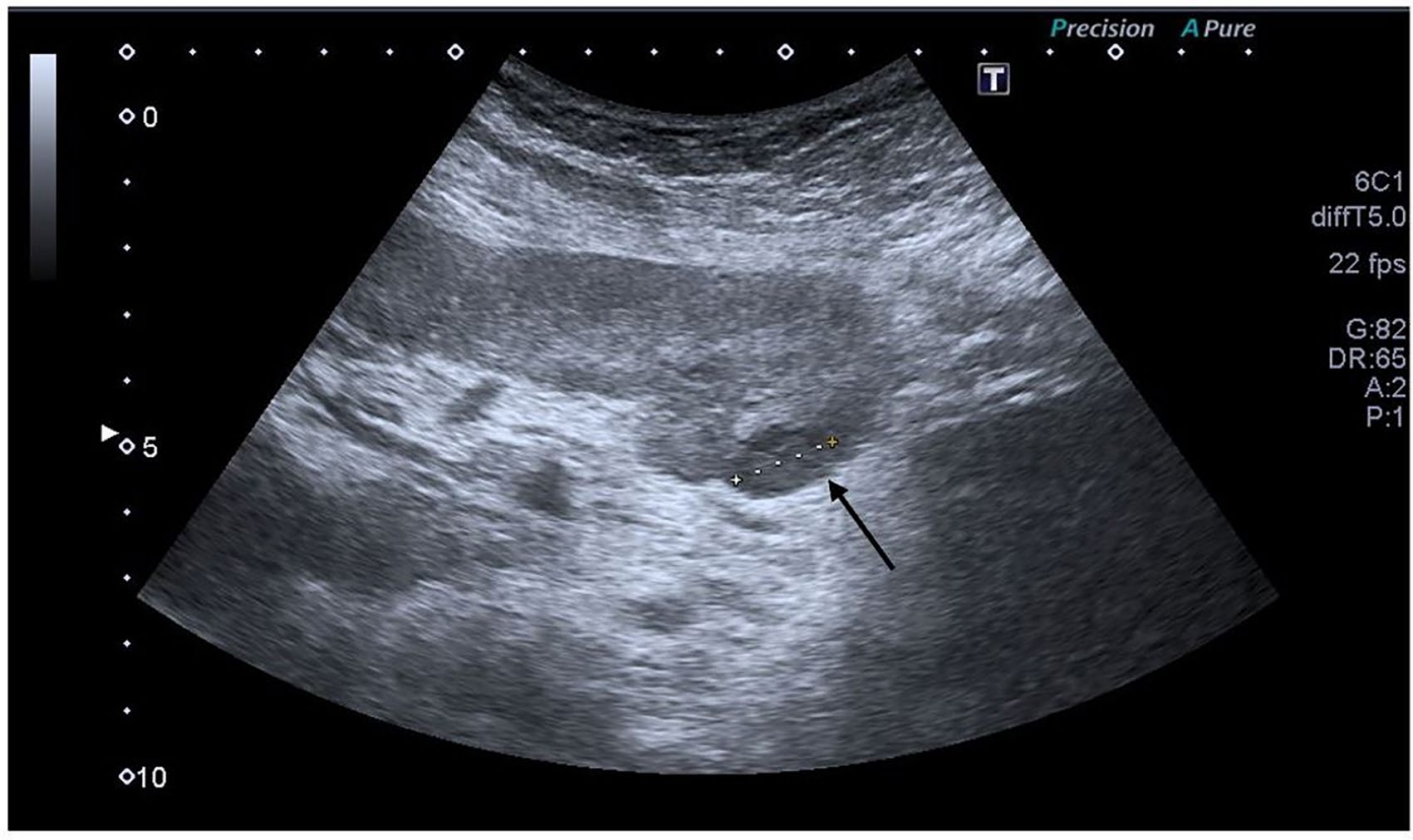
Ultrasound of the growing formation (arrow) in the kidney graft seen in the left kidney transplant recipient. An aspiration needle biopsy showed poorly differentiated cells of metastatic adenocarcinoma.
